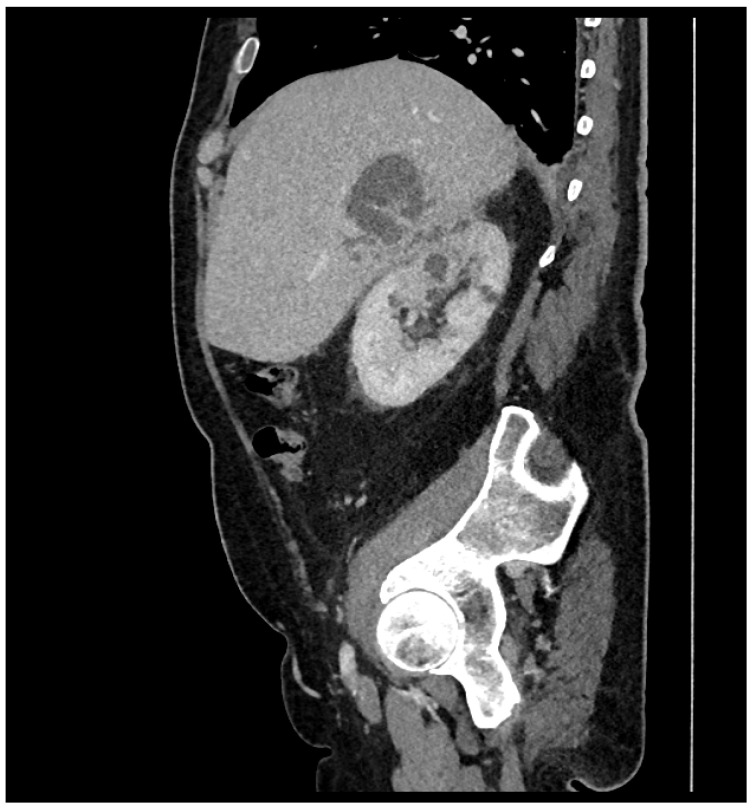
Abdomen CT scan. Sagittal section of the first abdominal CT scan performed showing an abscessualised right pyelonephritis and an intra-hepatic abscess.
